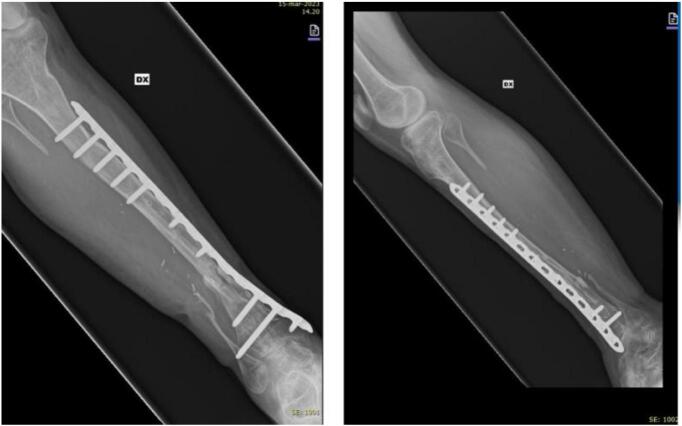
Radiographic outcome of the Huntington procedure.
